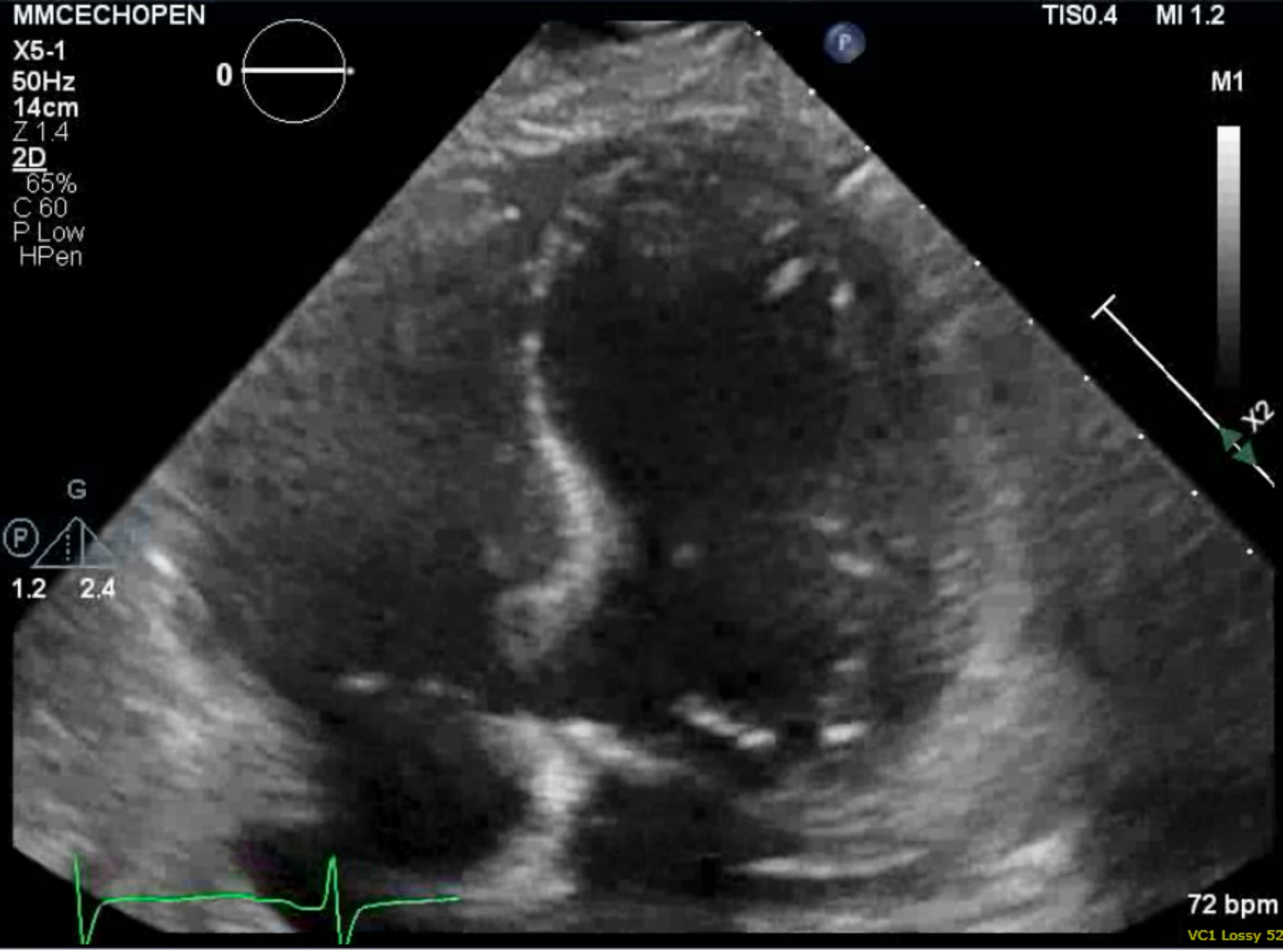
Transthoracic echocardiogram apical four chamber view showing left ventricular apical ballooning during systole.
